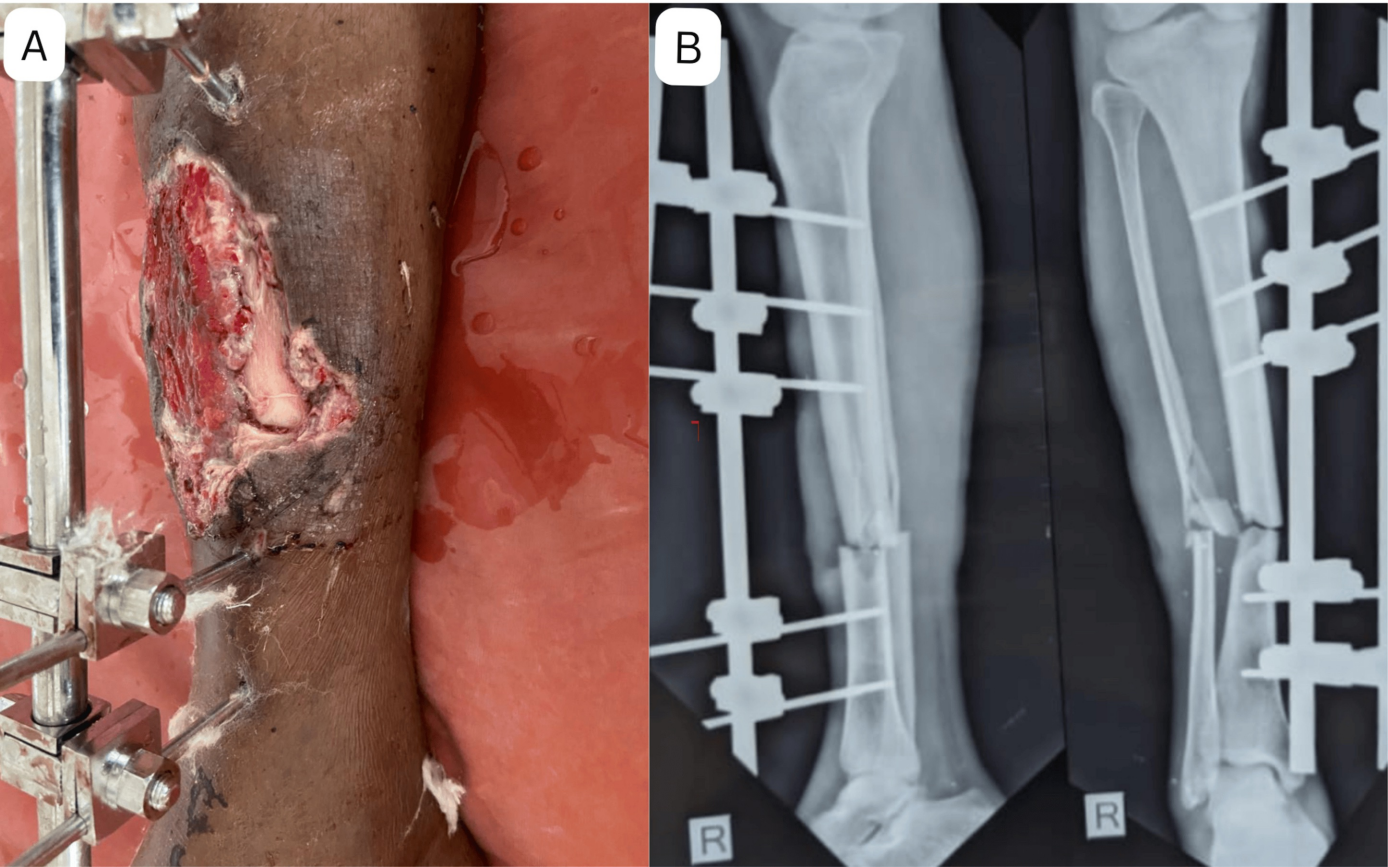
One-and-a-half-month old Gustilo-Anderson Type IIIB open tibial fracture on presentation. (A) Infected wound in mid third tibia, exposed tibia with gap at fracture site with AO tubular fixator in-situ. (B) Anteroposterior and lateral radiograph of both bones leg. These images correspond to Case 1 from the study.
